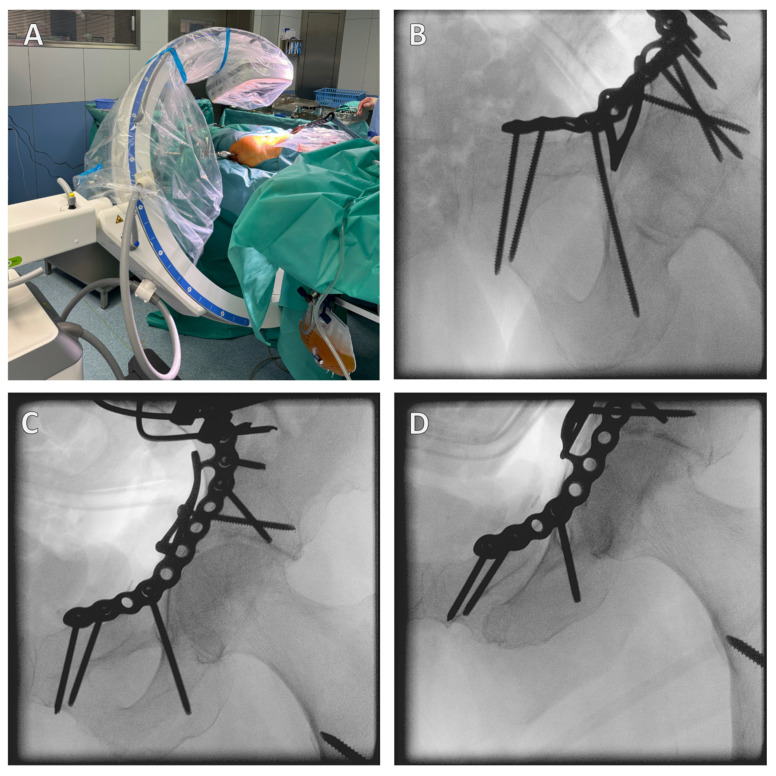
Intraoperative X-ray control. It is important to position the patient correctly; a carbon table should be used, and this should be moved out as far as possible so that there is plenty of space to swivel the X-ray C-arm (A). The correct screw position is checked in different planes. Here, the infra-acetabular screw is in the correct position, i.e., not through the joint (B–D).
